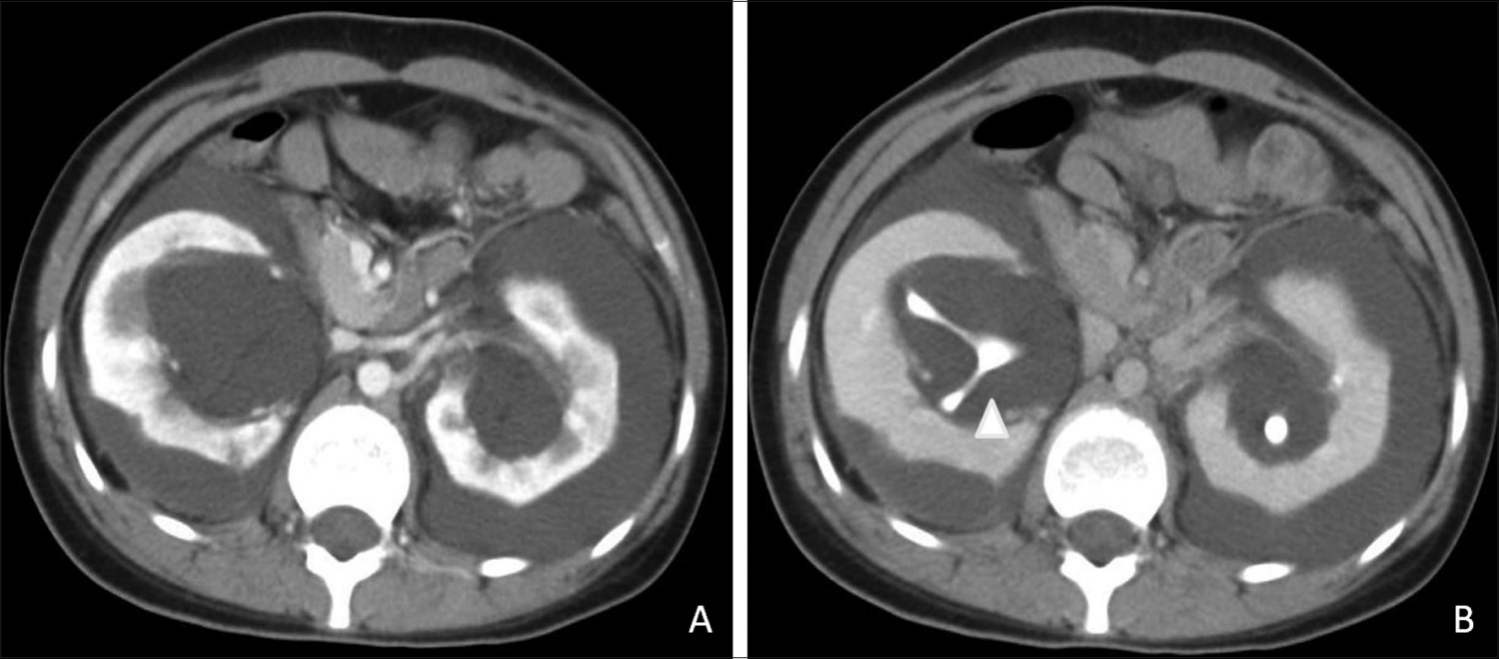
Contrast-enhanced CT scans in the corticomedullary (A) and excretory (B) phases show normal cortical enhancement with nonenhancing perirenal collections. The excretory images show splaying of the non-dilated excreting collecting system (arrowhead)
